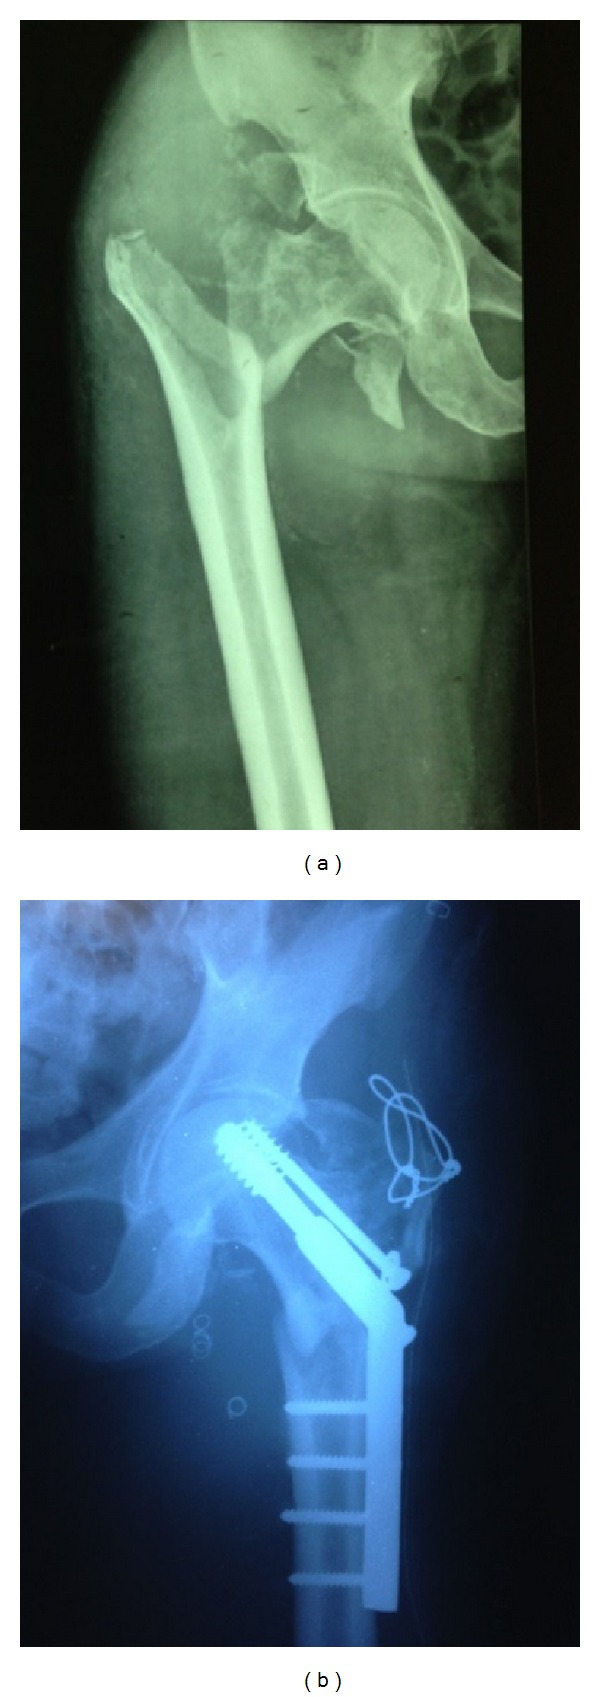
Conventional radiographs of fracture, coiling, and fixation. (a) Showing the inter trochanteric fracture with greater trochanter fracture right hip and (b) showing the endovascular repair done with coiling first and later dynamic hip screw (DHS) fixation and tension band wiring.
